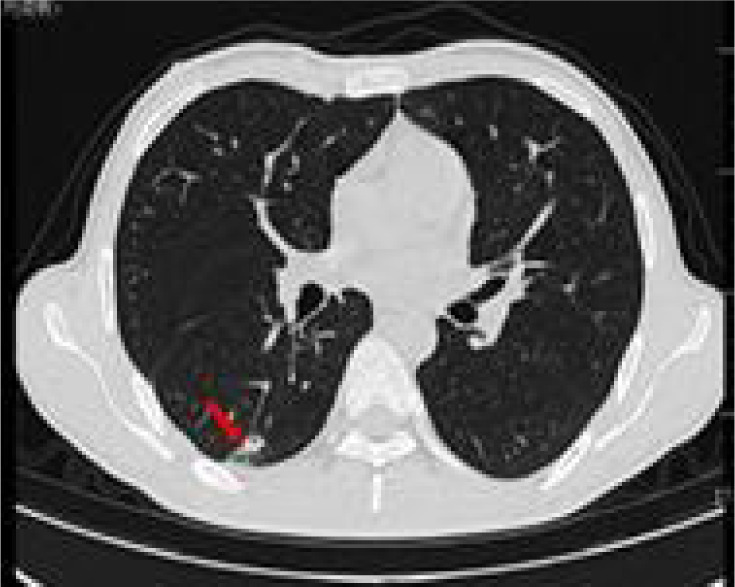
Lung CAT (CT) shows a ground glass nodule (12×9 mm) in the posterior basal segment of the right lower lobe (IM51), with signs of pleural depression (red arrow).
